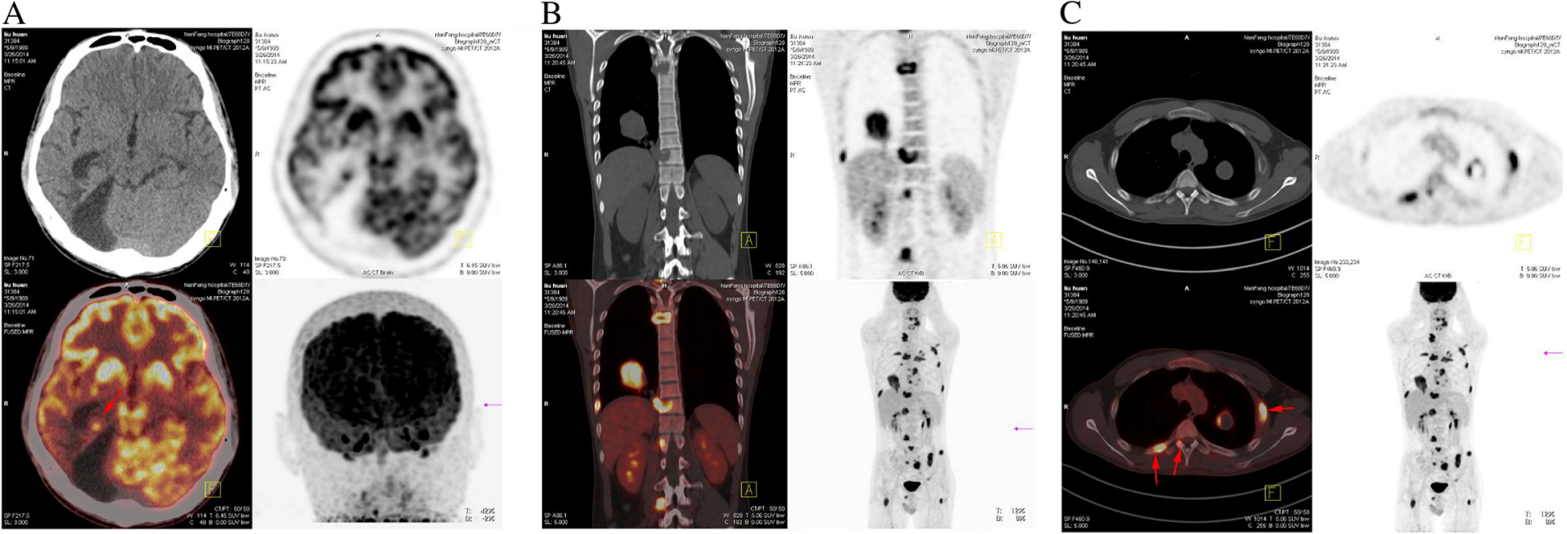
PET/CT findings upon admission. (a) Tumor recurrence of the right occipital lobe. (b) Hyper-metabolic foci at the lower right lung and the vertebrae. (c) Hyper- metabolic foci at the vertebrae and ribs
